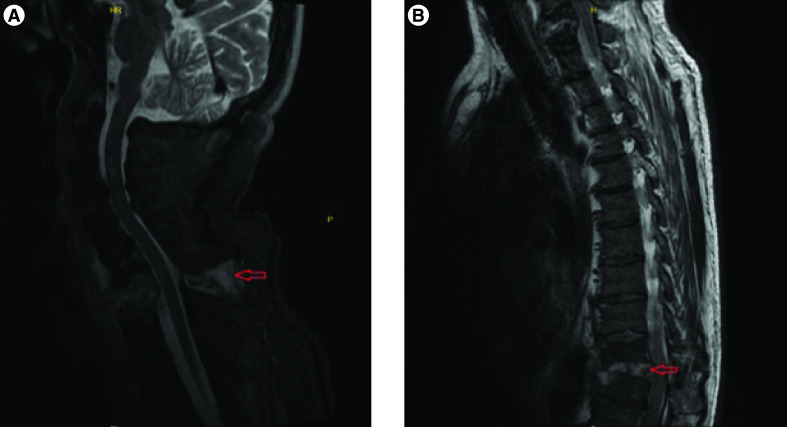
MRI thoracic and cervical spine with contrast. (A) Lesions compatible with epidural abscess are observed in C7–T1 bodies (arrow). (B) Lesions compatible with epidural abscess are observed in T10–11 bodies (arrow). C: Cervical spine; T: Thoracic spine.
